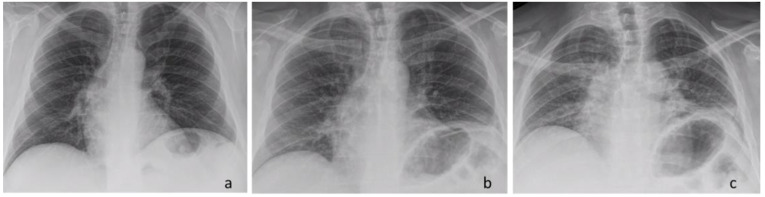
Chest X-ray: Pre-operative finding—inspiration view (a). Post-operative finding—inspiration view (b). Post-operative finding—expiration view (c).
